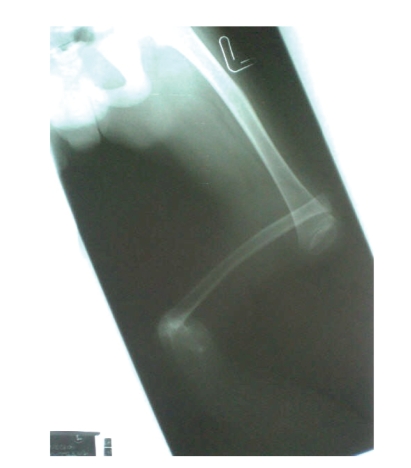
X-ray: complete agenesis of the left tibia, shortened and thickened fibula, and hypoplastic distal femoral epiphysis.
